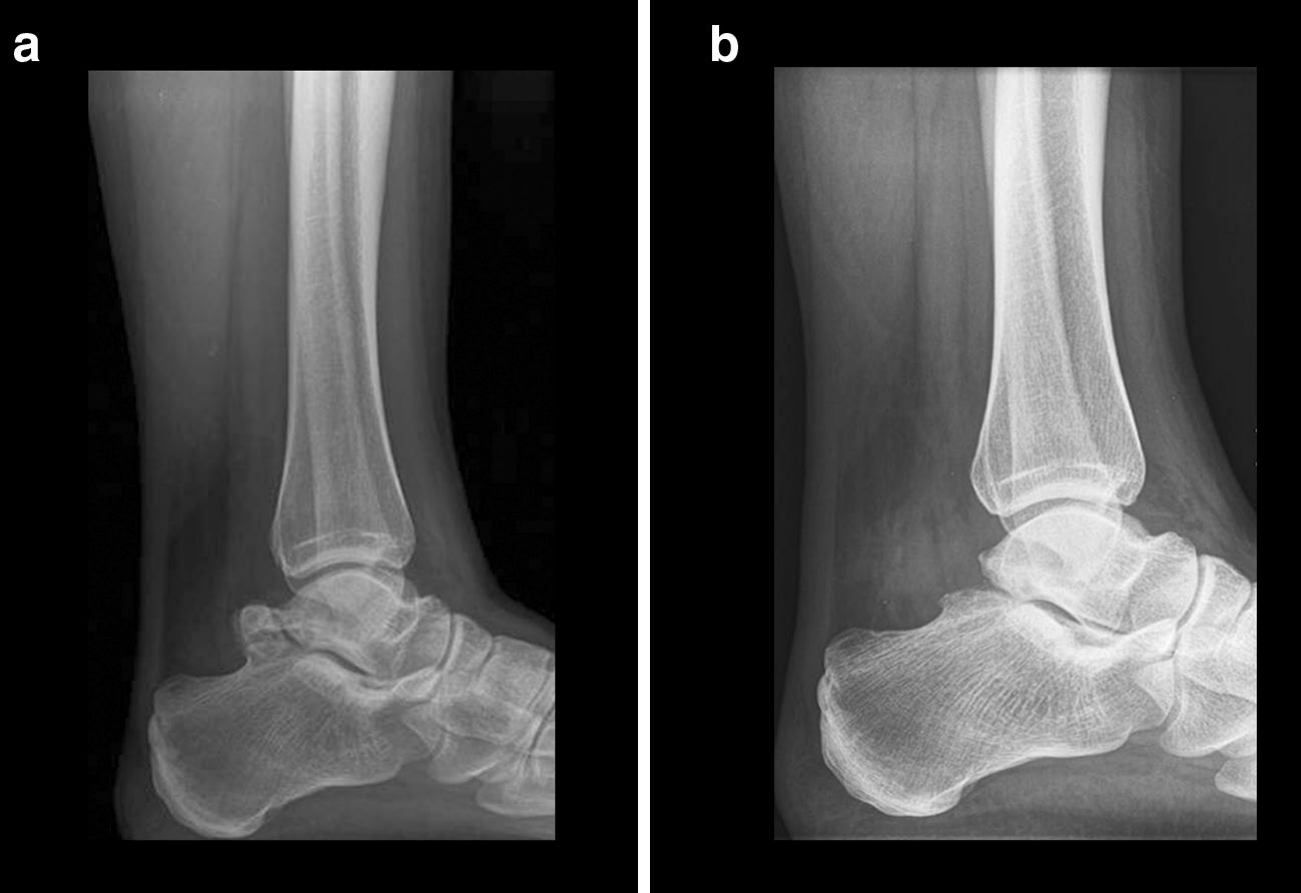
Lateral radiographs case 1. a Preoperative radiograph showing talus bipartitus. b Radiograph one year following surgery
